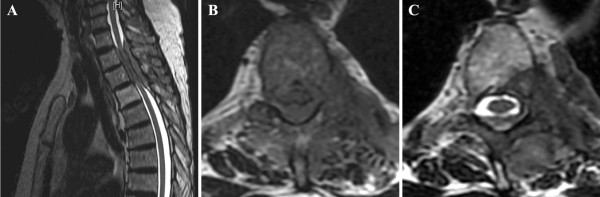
Imaging of the spine demonstrating the epidural tumor mass *.* A) Sagittal T2-weighted MRI showing the dorsal epidural lesion with cord compression. B) Axial T2-weighted MRI showing circumferential epidural compression of the spinal cord by the tumor mass with, C) tumor extension through the intervertebral foramen with lateral extension into the paraspinal tissues.
